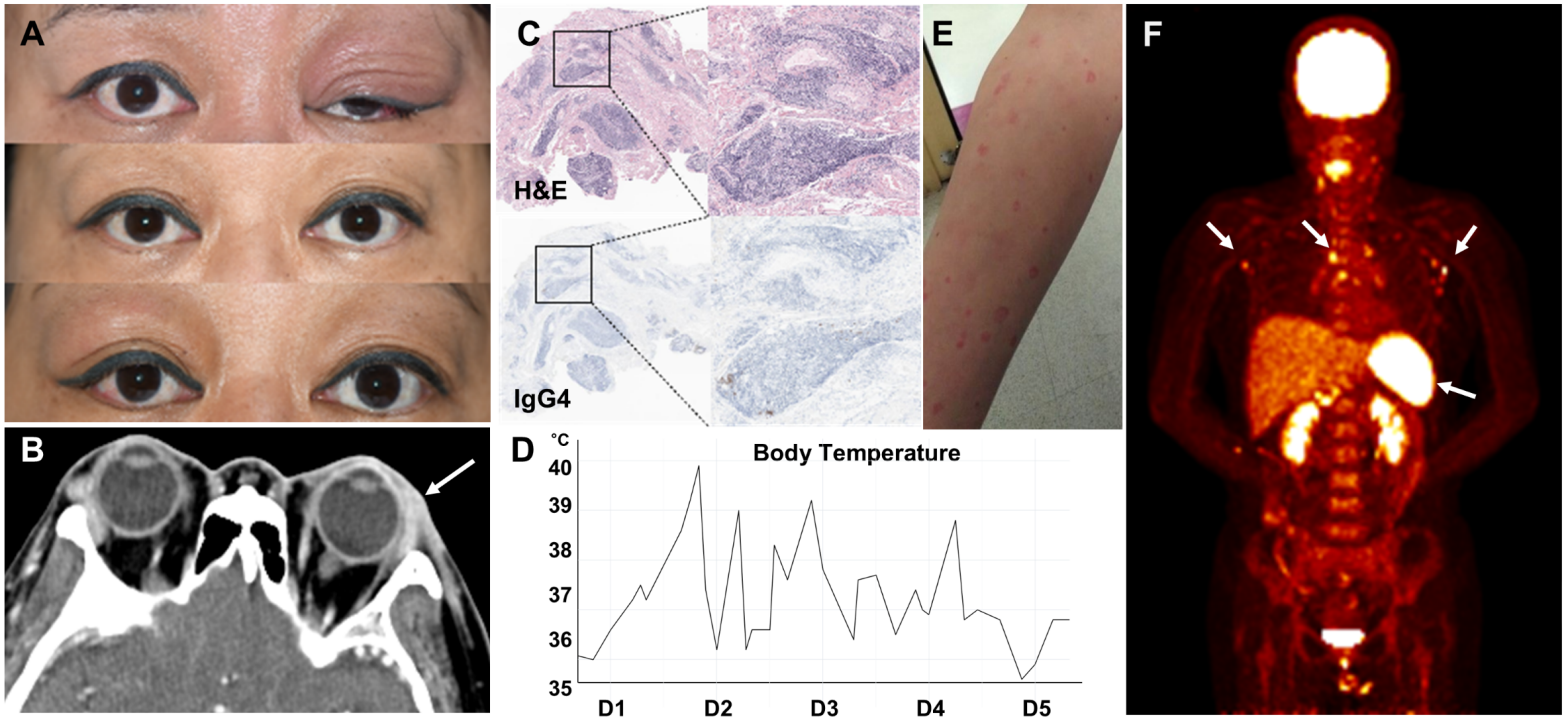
Recurrent periorbital swelling. (A) Recurrent and alternating swelling of the patient’s eyelids. (B) CT image of the orbital area showing soft tissue infiltration in the preseptal region, lacrimal gland, and left orbit (arrow). (C) Lacrimal gland biopsy showing multifocal lymphoplasmacytic infiltration (upper panel) and a few IgG4-positive cells (lower panel). (D) Temperature graph showing the patient’s fever pattern during the first 5 days of admission. (E) Maculopapular eruption. (F) PET scan showing increased metabolic activity in lymph nodes and spleen (arrows).
